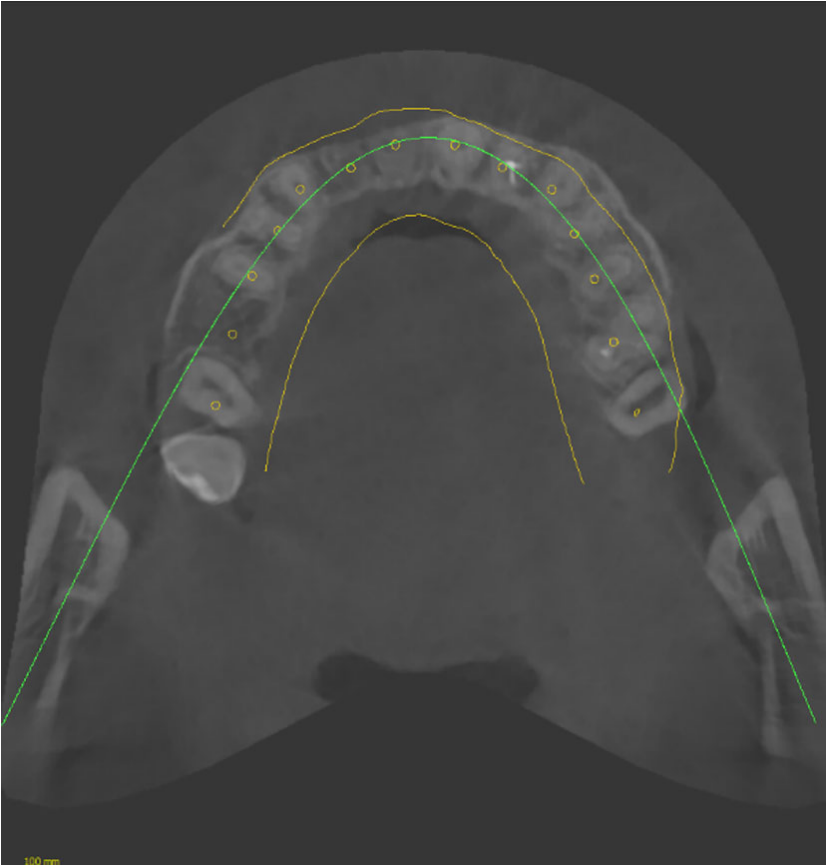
Using CoDiagnostix, a superimposition of the CBCT-scan with the axes reconstructed by the SSM and a dental wax up (both in yellow) was performed. The axes calculated by the SSM were depicted as yellow cylinders. Therefore, in this horizontal cross section, they appear as a dot. The yellow dots mostly match with the hypodense area of the root canals of the teeth (if existent)
